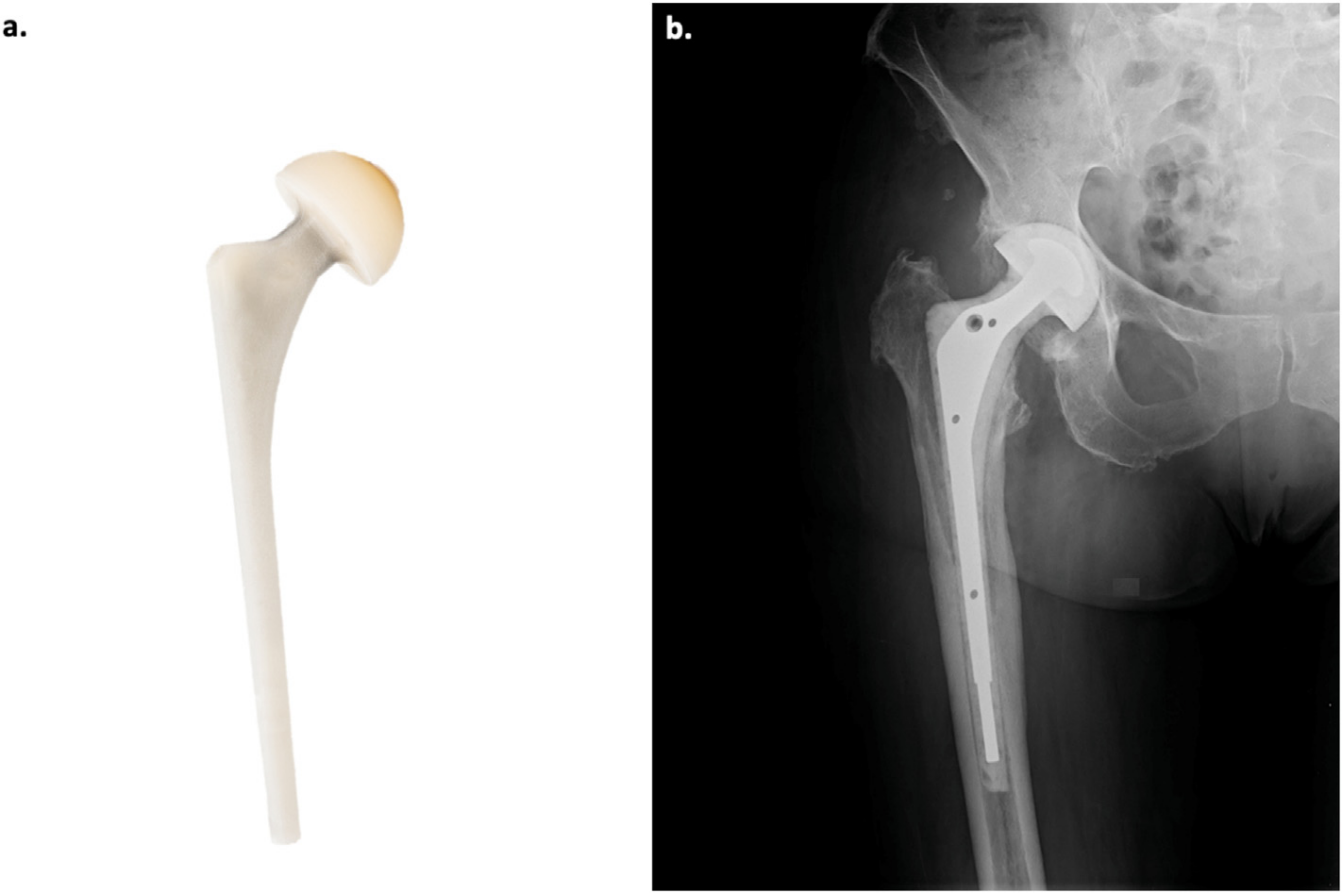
Fig. 3a: 3D model of the Spaceflex hip G21 spacer. Fig. 3b: An anteroposterior (AP) X-ray of the right hip showing an implanted Spaceflex in the immediate postoperative during the interval period of a two-stage revision.
